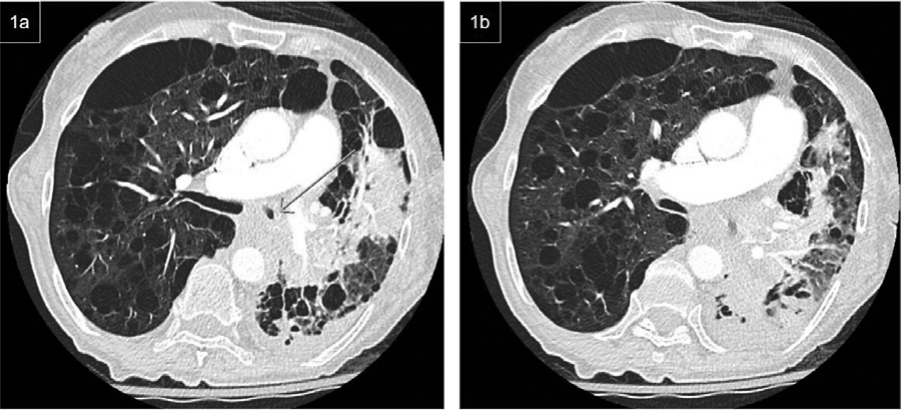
(A, B) Axial CT image shows infiltrate and postobstructive atelectasis in the left lower lobe with stenosis and debris in the left mainstem bronchus. Soft tissue attenuation around the mainstem bronchus raised suspicion for mass.
